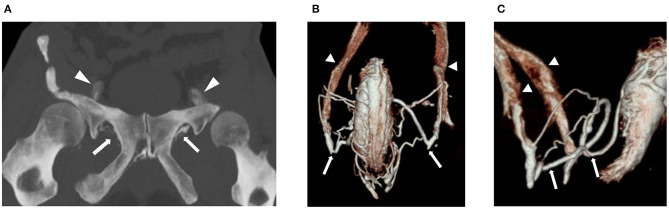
55-year old man with erectile dysfunction due to veno-occlusive disease. Patient is non-responder to PDE-5-inhibitor and Caverject. (A) Contrast enhanced CT cavernosography (coronal maximum intensity projection) demonstrates bilateral venous leaks via pudendal veins (arrows) draining into iliohypogastric veins (arrowheads). (B,C) Contrast enhanced CT cavernosography (volume rendering in coronal (B) and sagittal (C) views) demonstrating bilateral venous leaks via pudendal veins (arrows) draining into iliohypogastric veins (arrowheads).
